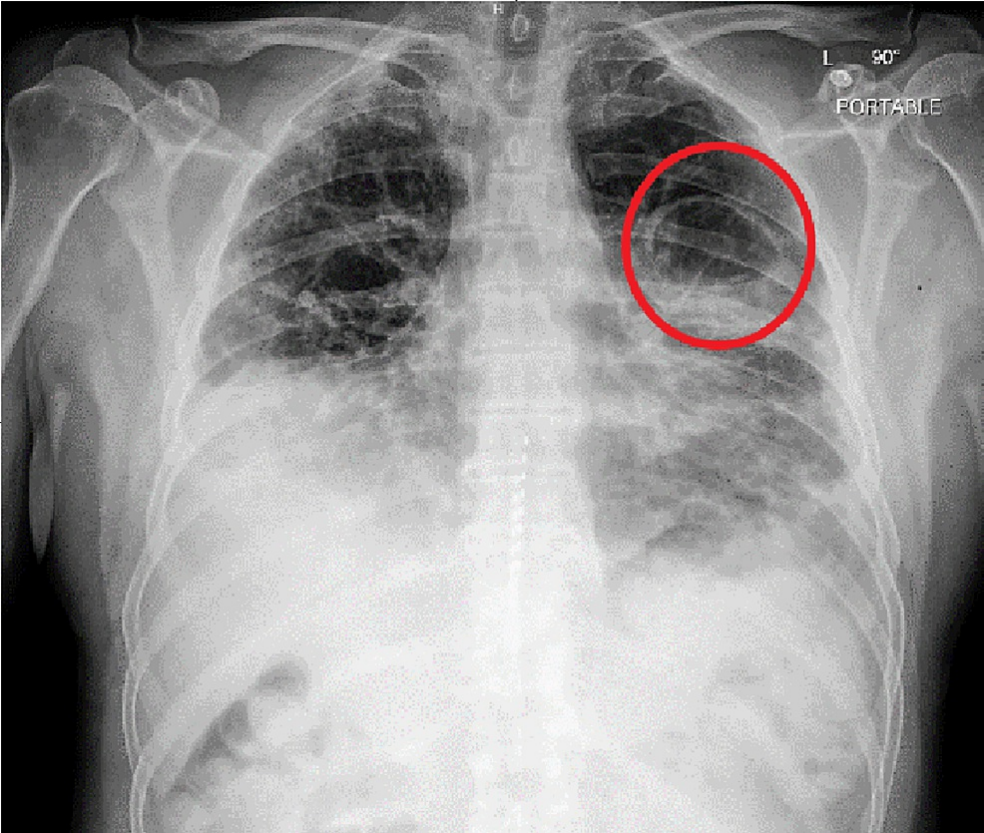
Chest X-ray of pneumatocele in left upper lobe of lung (red circle)
